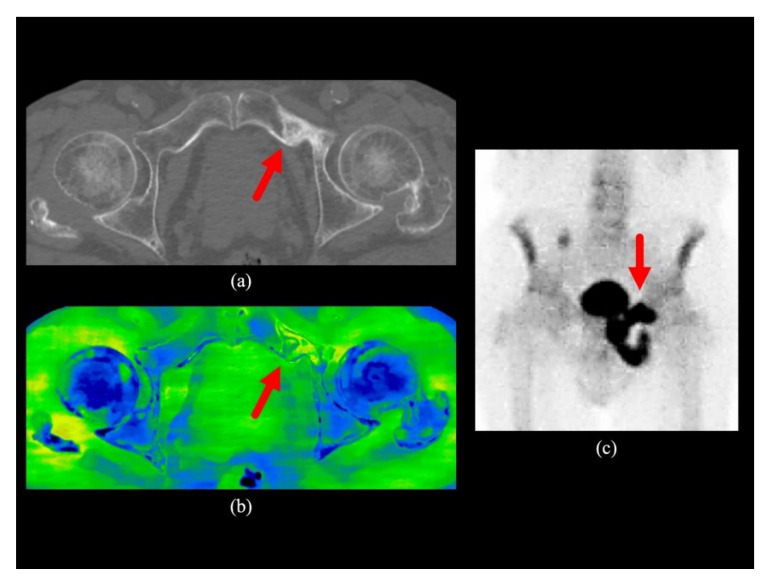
Clinical case 1. A man in his 80s with bone metastasis of prostate cancer. (a) The transaxial image of the conventional CT of the pelvis shows the sclerotic bone metastasis in the left superior pubic branch; (b) The transaxial water-HAP image of the pelvis shows a localised increase in water density compared to the other side (red arrow); (c) The anterior view of the bone scintigraphy shows increased uptake in the same region (red arrow). HAP; Hydroxyapatite.
